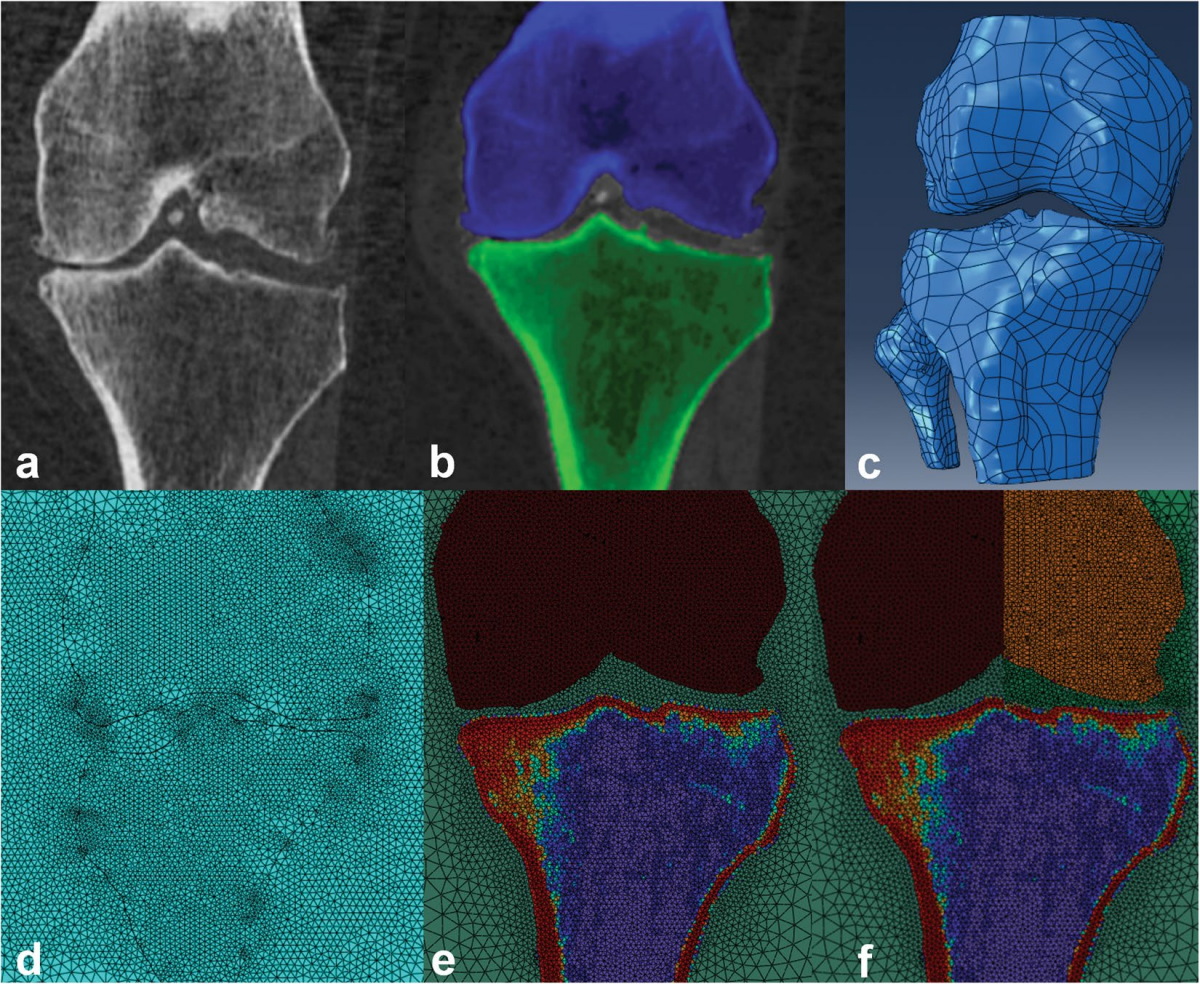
Methodological sequence for developing subject-specific FE model. (a) CT image of the knee. (b) Segmented bones of the knee. Image shows femur (blue) and tibia (green) in a coronal view. (c) Generated three-dimensional geometries of the femur, tibia, and fibula from CT images. (d) Meshed bones of the knee with 10-noded tetrahedral elements. Image shows femur, tibia, and soft tissue cylinder in the coronal plane. (e) Assigned material properties for the FE model. BMD was mapped to the modulus of elasticity of the bones. (f) To calculate the stiffness of medial compartment of the proximal tibia, the lateral compartment was isolated by assigning soft tissue material properties to the lateral distal femur.
